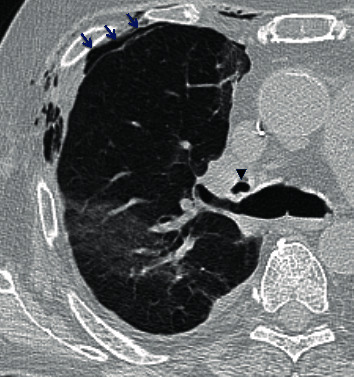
Axial chest CT showed a sentinel pocket of gas adjacent to the right bronchial anastomotic site (black caret); an anterior pneumothorax (blue arrows) is also seen.
